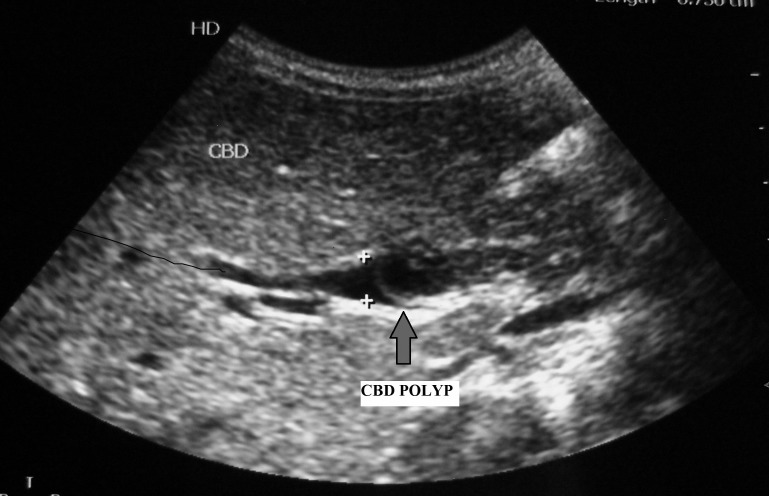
Figure 1:Ultrasound showing filling defect in the lower CBD (polyp).
